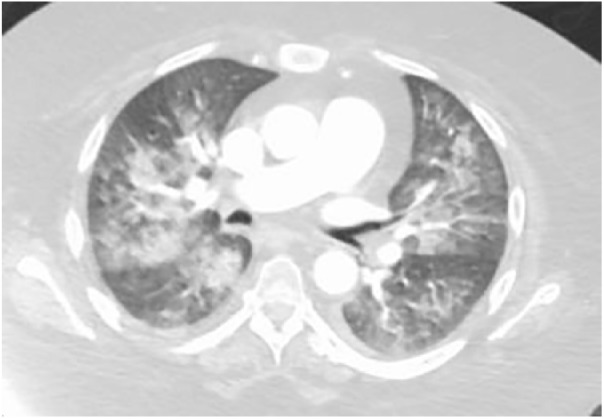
Computed tomography scan of chest with contrast with bilateral diffuse alveolar infiltrates and pleural effusions on hospital day 2.
